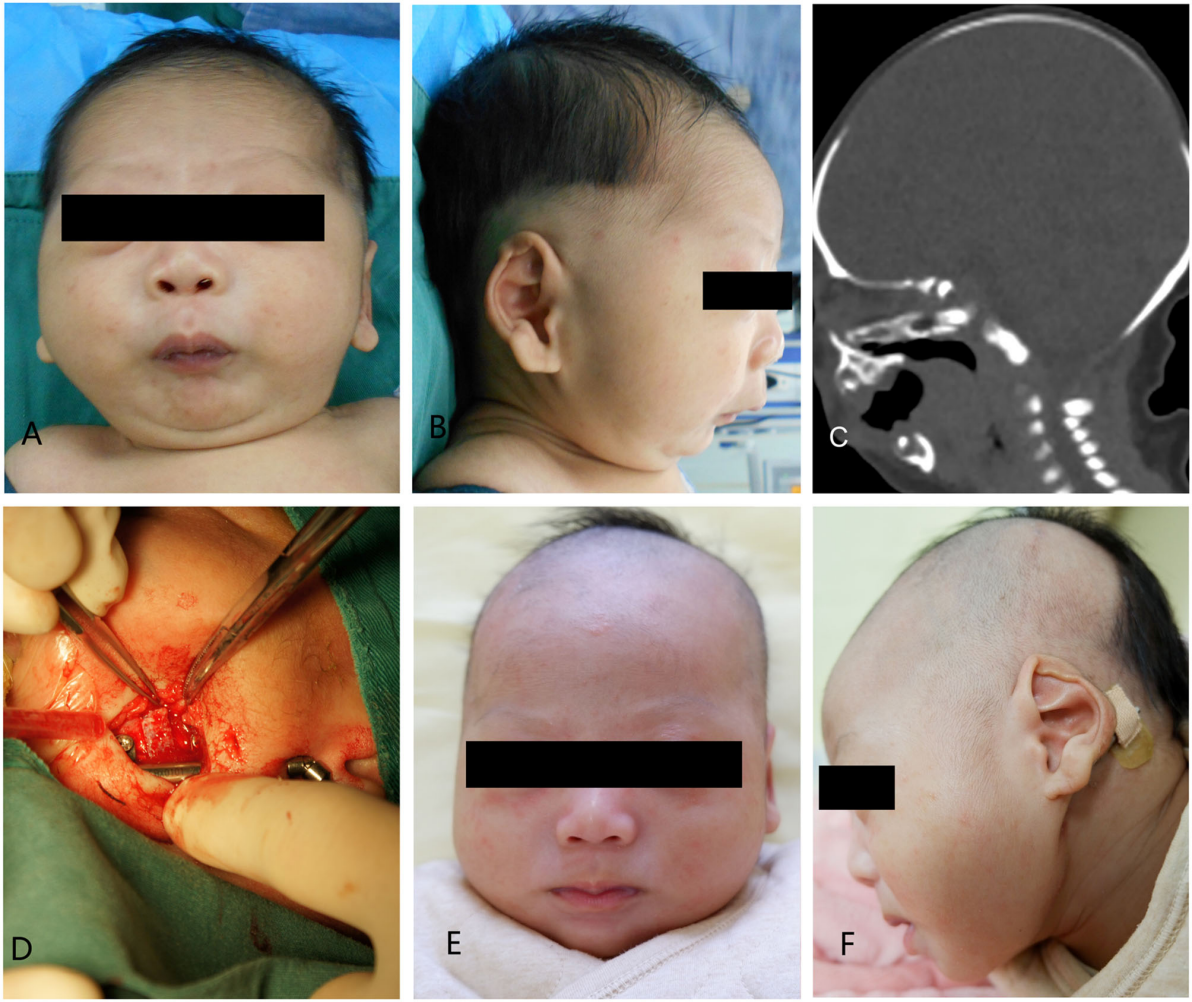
Case 1. (A) A preoperative frontal view. (B) Preoperative lateral view. (C) Preoperative CT-scan. (D) Intraoperative situation. (E) Postoperative anteroposterior view. (F) Postoperative lateral view.
